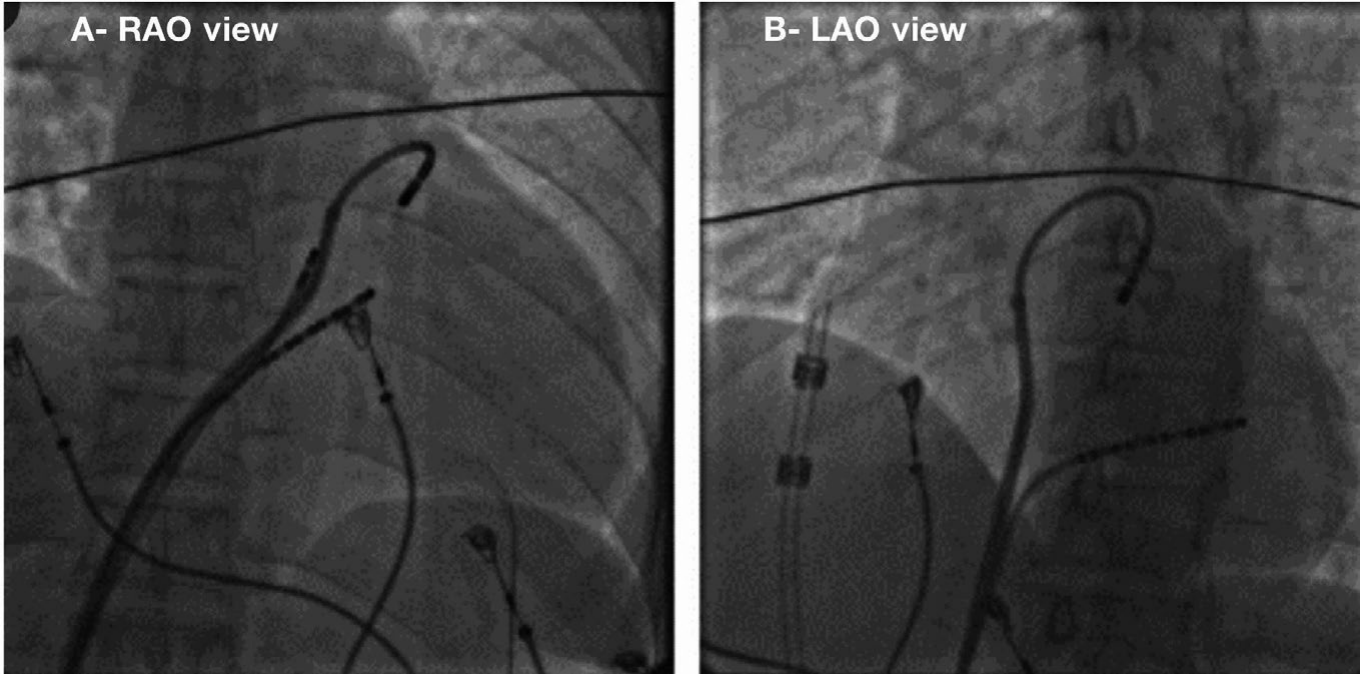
Right anterior oblique (RAO) and left anterior oblique view (LAO) radiographic view of mapping catheter in inverted U shape in anterior pulmonary cusp
